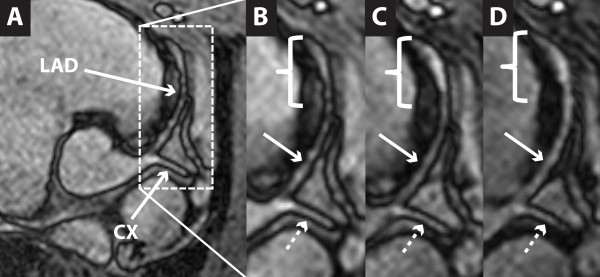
Coronary bright-blood CMR-angiography (A). T1-weighted inversion recovery at 5 min (B) 15 min (C), and 25 min (D) following intravenous administration of gadofosveset. The area of the balloon injured LAD2 segment expands over time indicating time-dependent extravasation of contrast, whereas the intact LAD1 segment (arrow) and CX (dotted arrow) remain constant.
